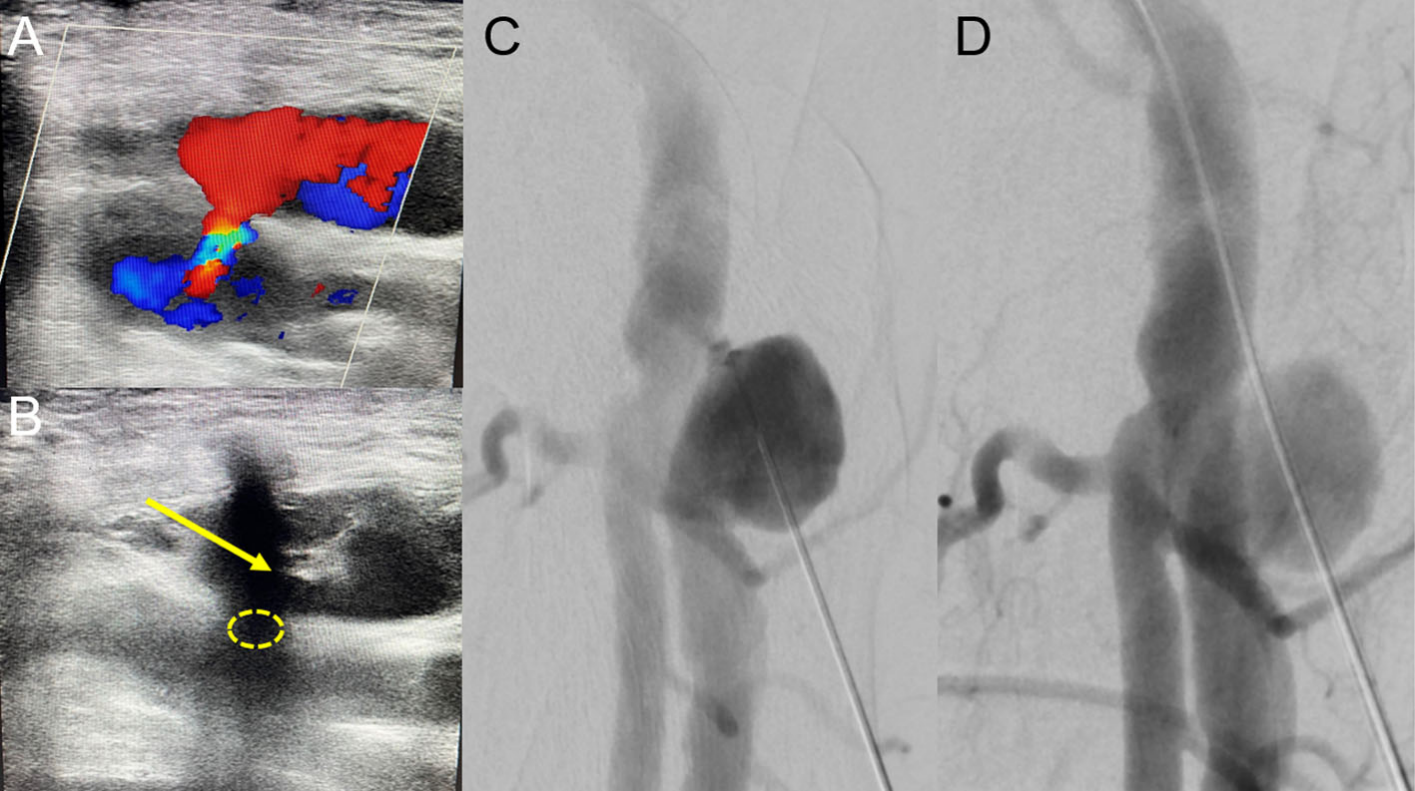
Huge PSA in a 94-year-old patient (Patient No. 2) after TAVI and a successfully performed TA maneuver on day 6 after the intervention. A Color-coded duplex sonography showing a huge PSA with a narrow neck and strong and turbulent perfusion. B Puncturing of the PSA with the needle tip (yellow arrow) close to the neck (dashed yellow circle). C Consistent with ultrasound (B), DSA confirms direct puncture of the PSA neck. D Despite successful and correct insertion of the 5F sheath through the PSA neck, there is persistent perfusion of the PSA. Nevertheless, sonography on the next day shows complete absence of perfusion from the PSA following the TA maneuver
